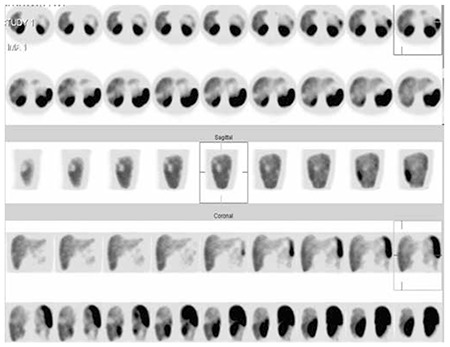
Technetium-99m-labeled octreotide acetate scintigraphy in the SPECT mode. It revealed some photopenic regions in the liver, compatible with the hepatic masses on computed tomography images
